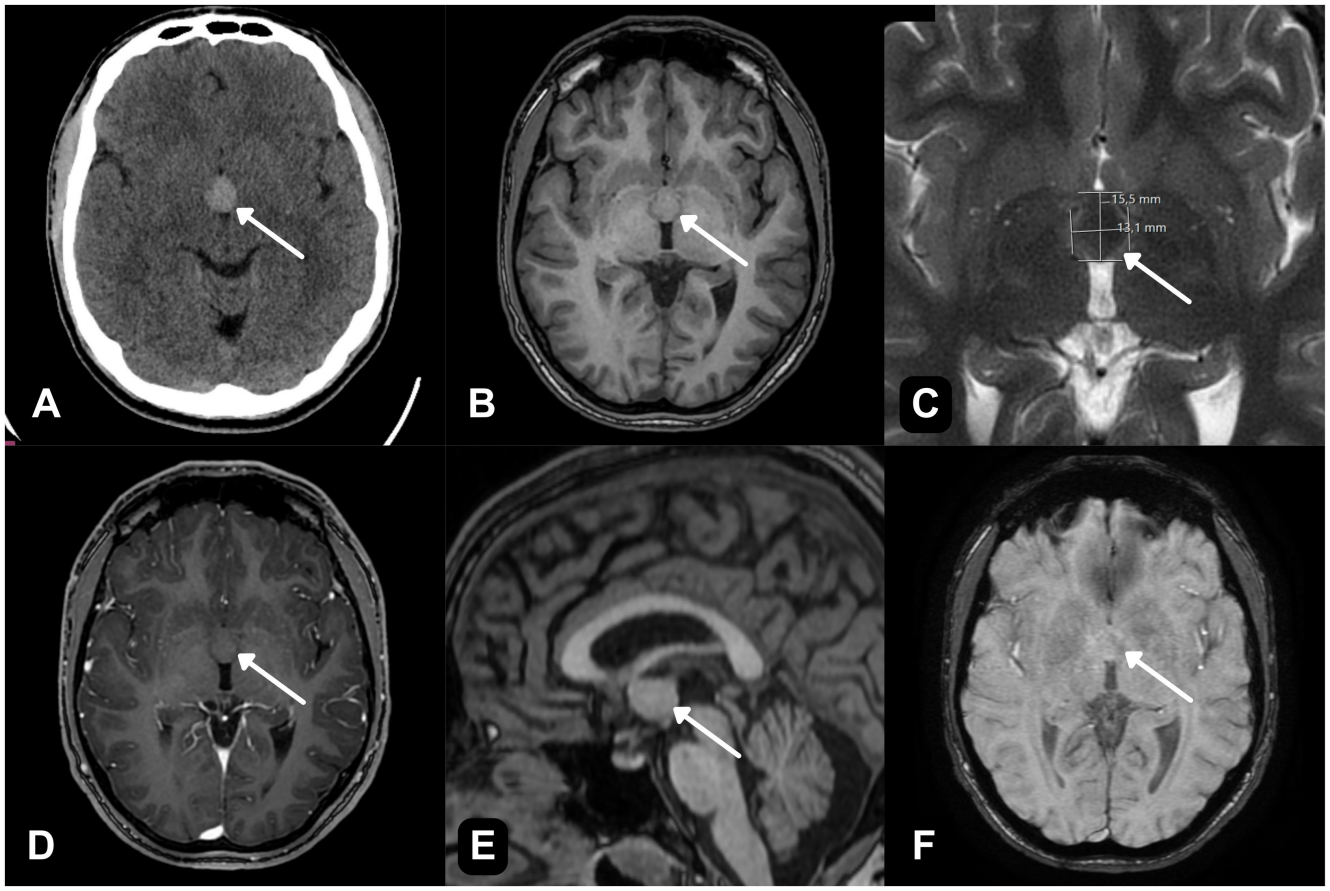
Initial CT and 1.5-T MR imaging. (A) Preoperative non-contrast CT demonstrates a hyperdense, well-defined intraventricular mass at the foramen of Monro (arrow). (B) Axial T1-weighted image shows a well-circumscribed lesion isointense to adjacent brain parenchyma within the anterior third ventricle (arrow). (C) Axial T2-weighted fast spin echo (FSE) image demonstrates a centrally hypointense area with a relatively hyperintense rim (arrow). (D) Axial post-contrast T1-weighted image shows no measurable enhancement (arrow). (E) Sagittal T1-weighted image confirms the lesion’s intraventricular location at the foramen of Monro (arrow). (F) SWAN/SWI shows no susceptibility to suggest intralesional hemorrhage or calcification (arrow).
